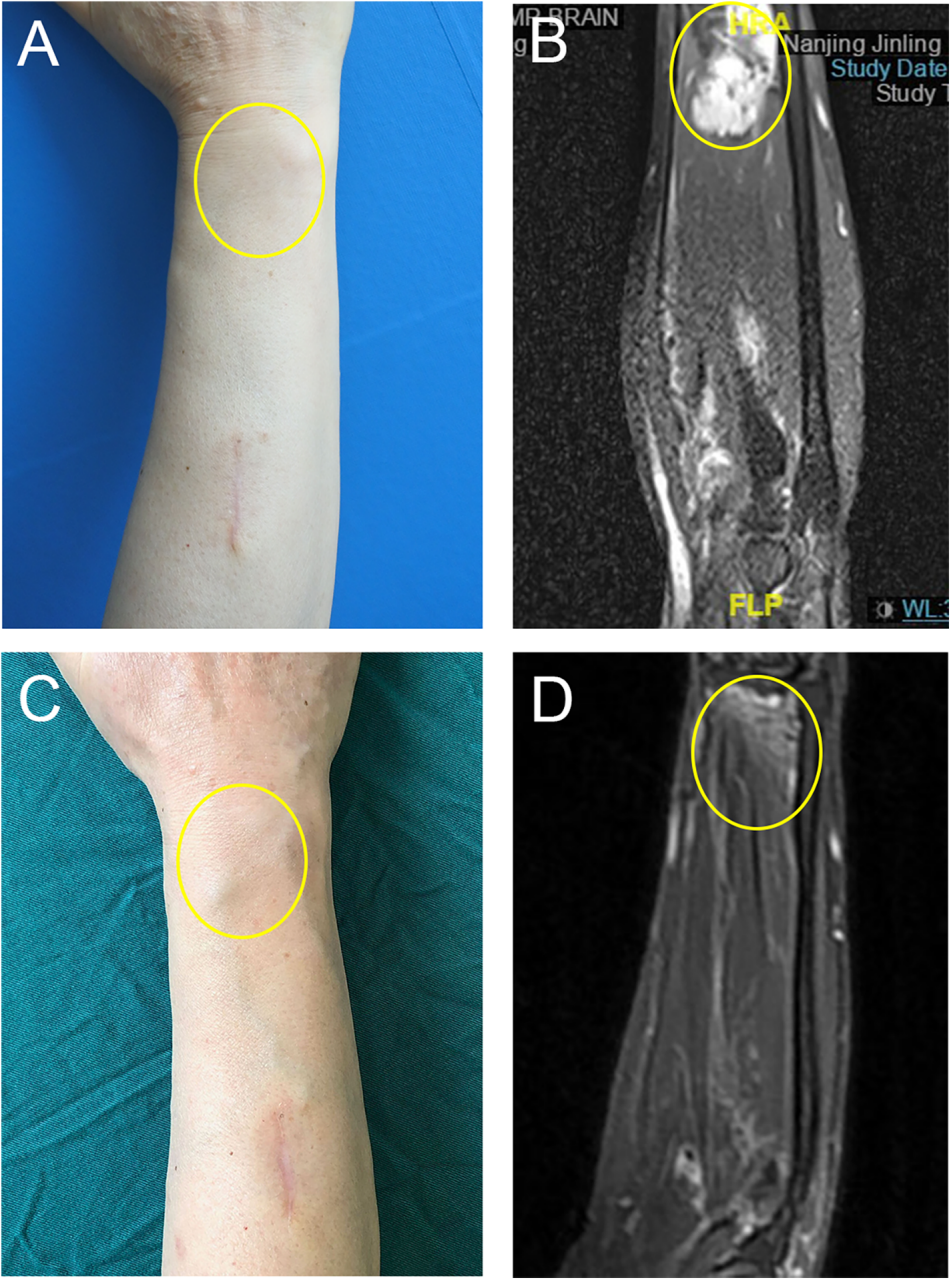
Focal mass type IMVM in the right upper extremity. (A) The appearance before treatment. (B) The MRI before treatment. (C) The appearance after three times of treatment. (D) The MRI after treatment. The yellow ellipse indicated the lesion. The copyrights of the figures were held by corresponding author.
